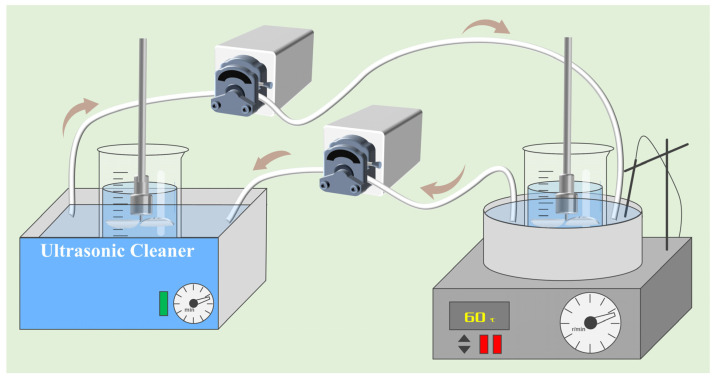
Experimental setup for ultrasound-assisted and conventional adsorption.
